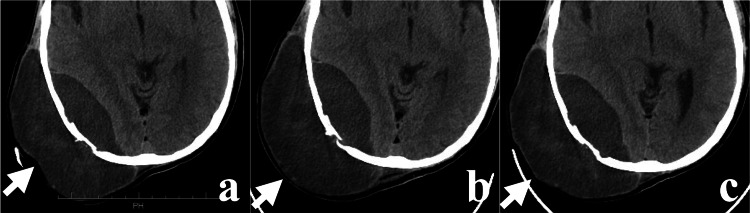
The change of cranial lesion swelling with or without DEX (white arrows) Plain axial CT showing the surface of the cranial lesion: (a) shrunk with oral administration of DEX 4 mg/day; (b) expanded without DEX; (c) re-shrunk with intravenous administration of DEX 6.6 mg/day. DEX: dexamethasone; CT: computed tomography.
